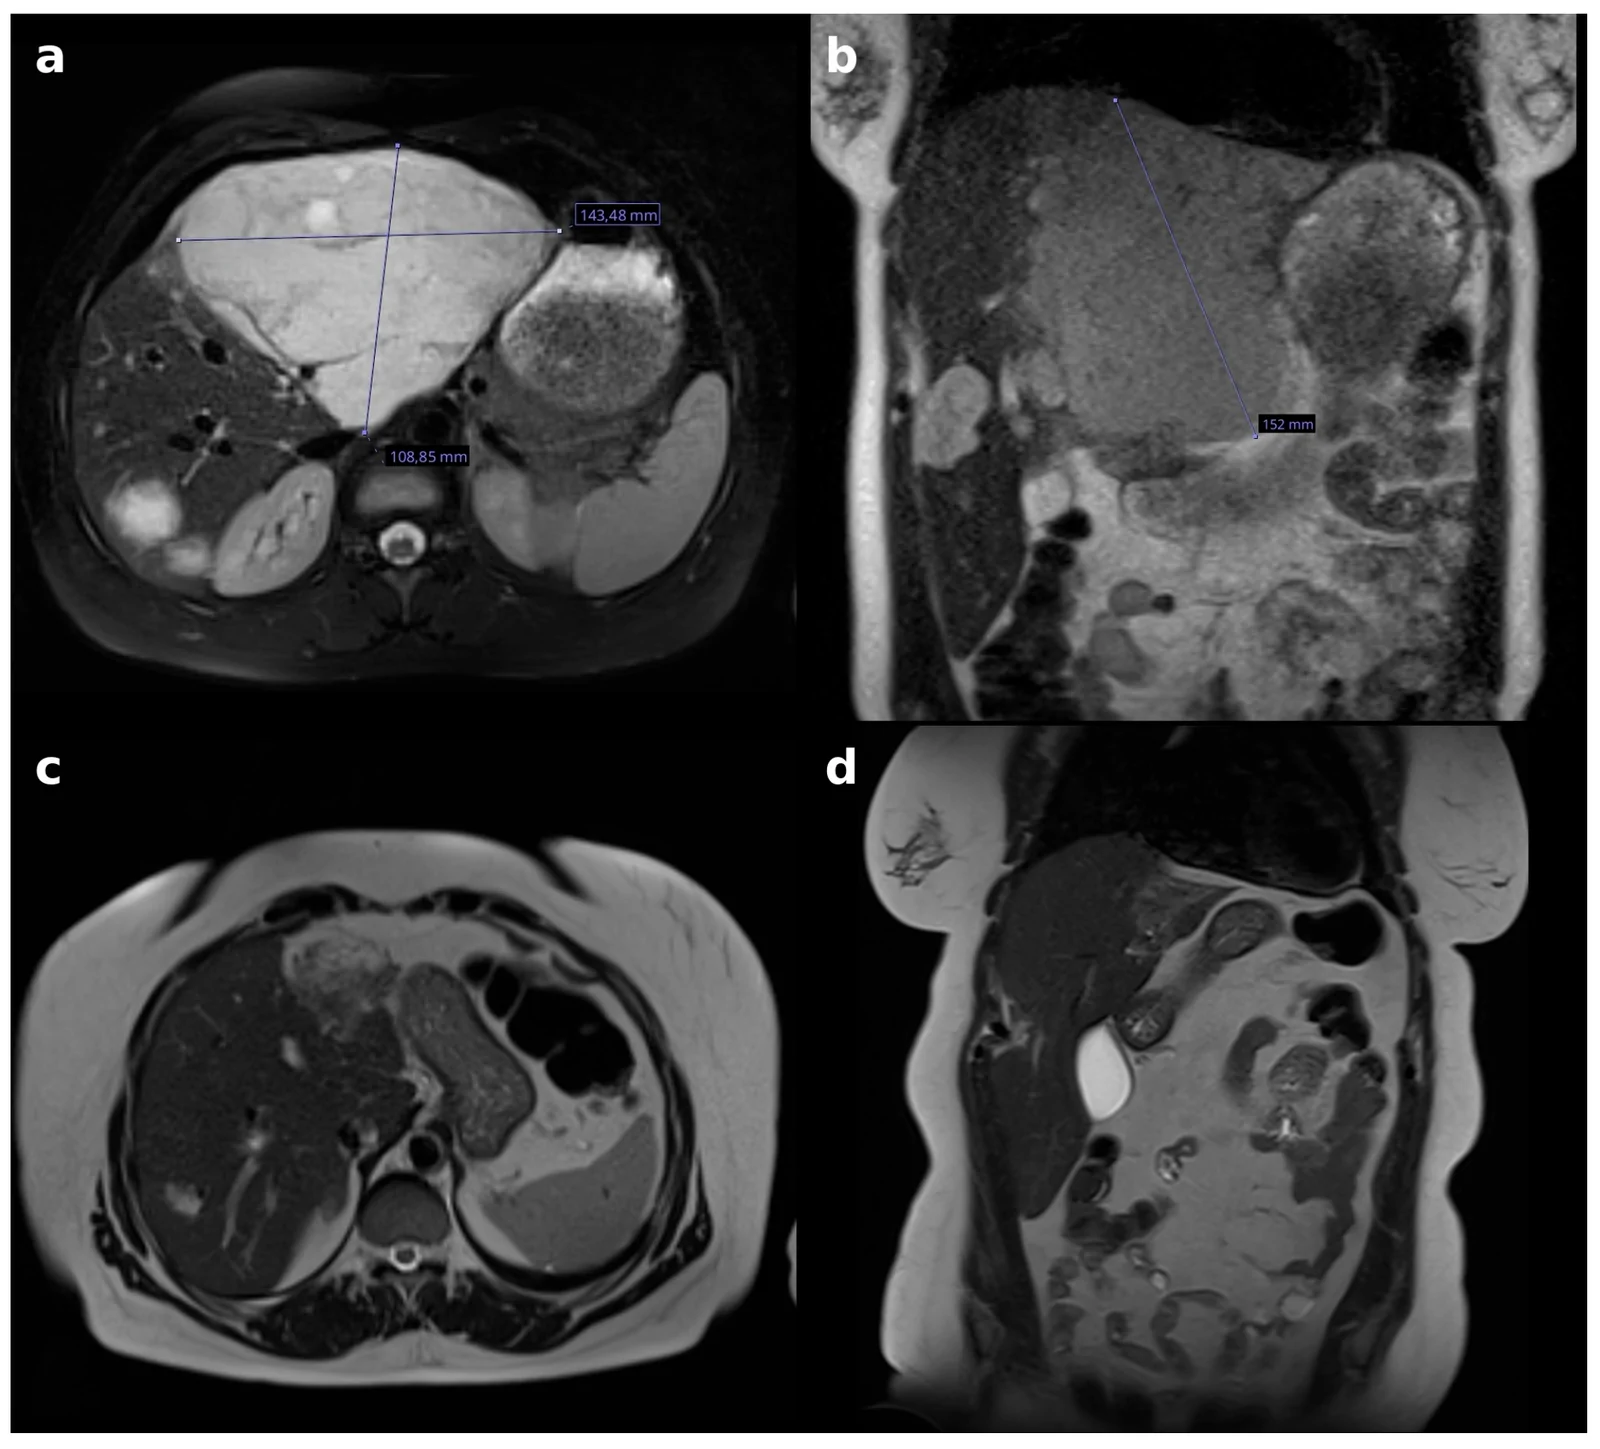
Pre- and post-embolization magnetic resonance imaging of a giant hemangioma of the left hepatic lobe. (a) Axial and (b) coronal T2-weighted images before embolization show a lesion measuring 15.2 × 14.3 × 10.9 cm. (c) Axial and (d) coronal images at the last follow-up, 85 months after embolization, show marked regression of the lesion to 5.5 × 4.3 × 3.9 cm (96% volume reduction).
